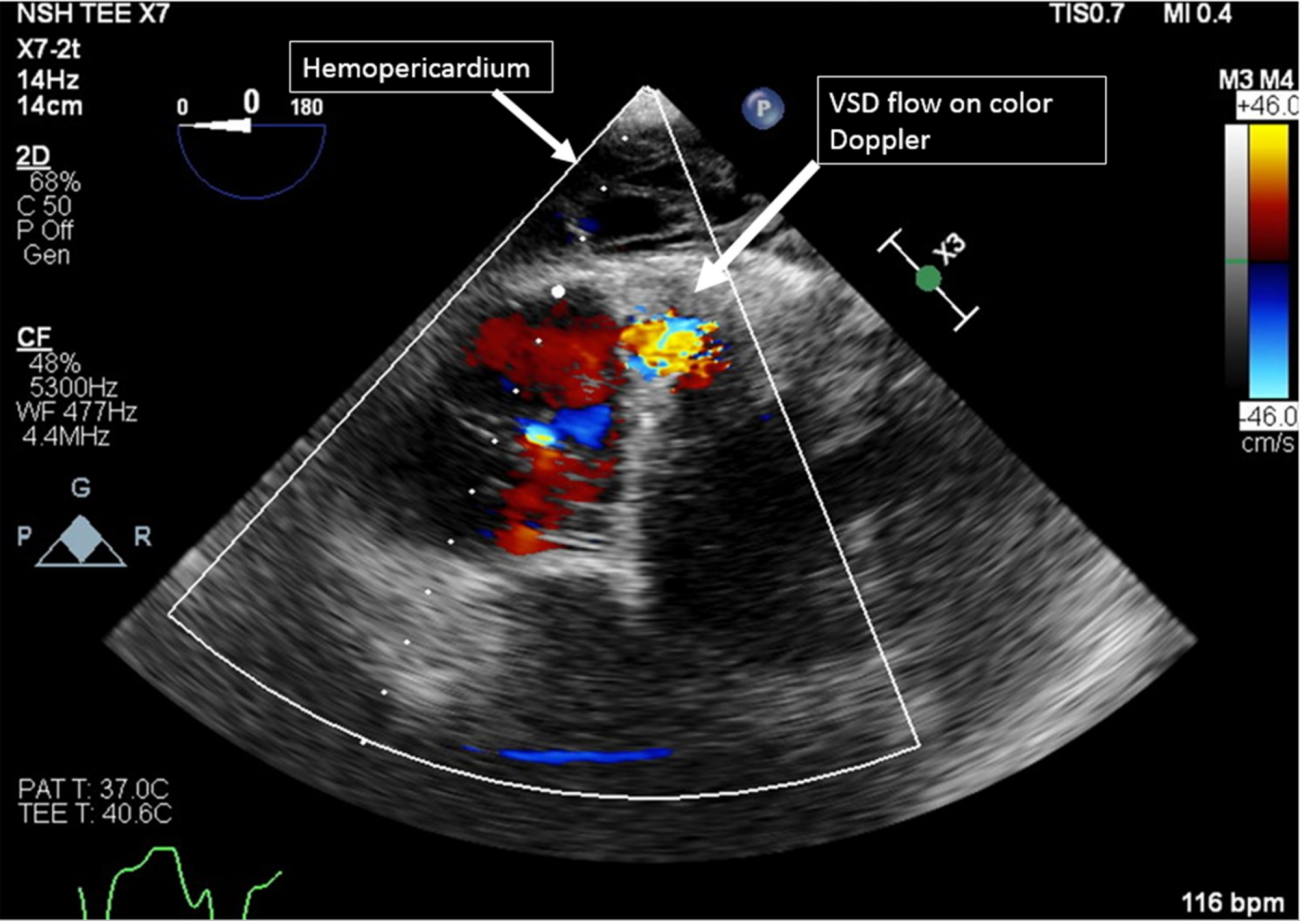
Transesophageal echocardiography (TEE) image of hemopericardium VSD: ventricular septal defect
